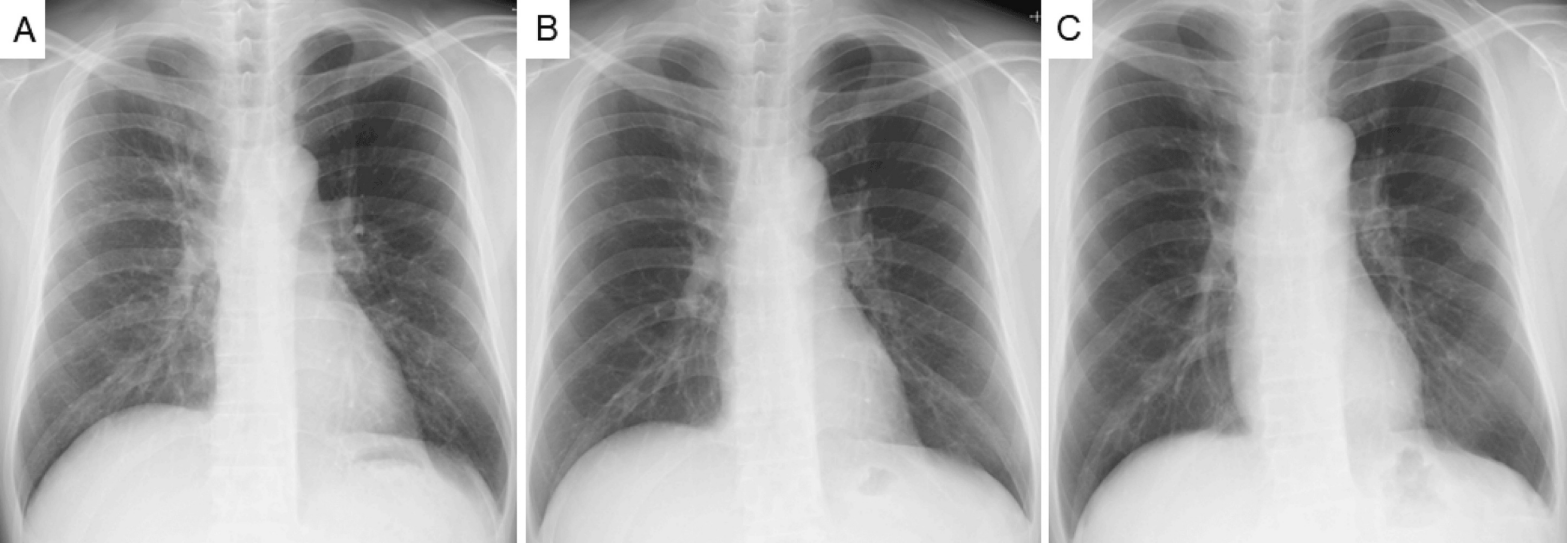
Serial chest X-rays demonstrating sustained long-term improvement after bronchoscopic mucus plug removal. (A) Chest X-ray obtained one week after bronchoscopy showing early improvement in bronchovascular bundle thickening.
